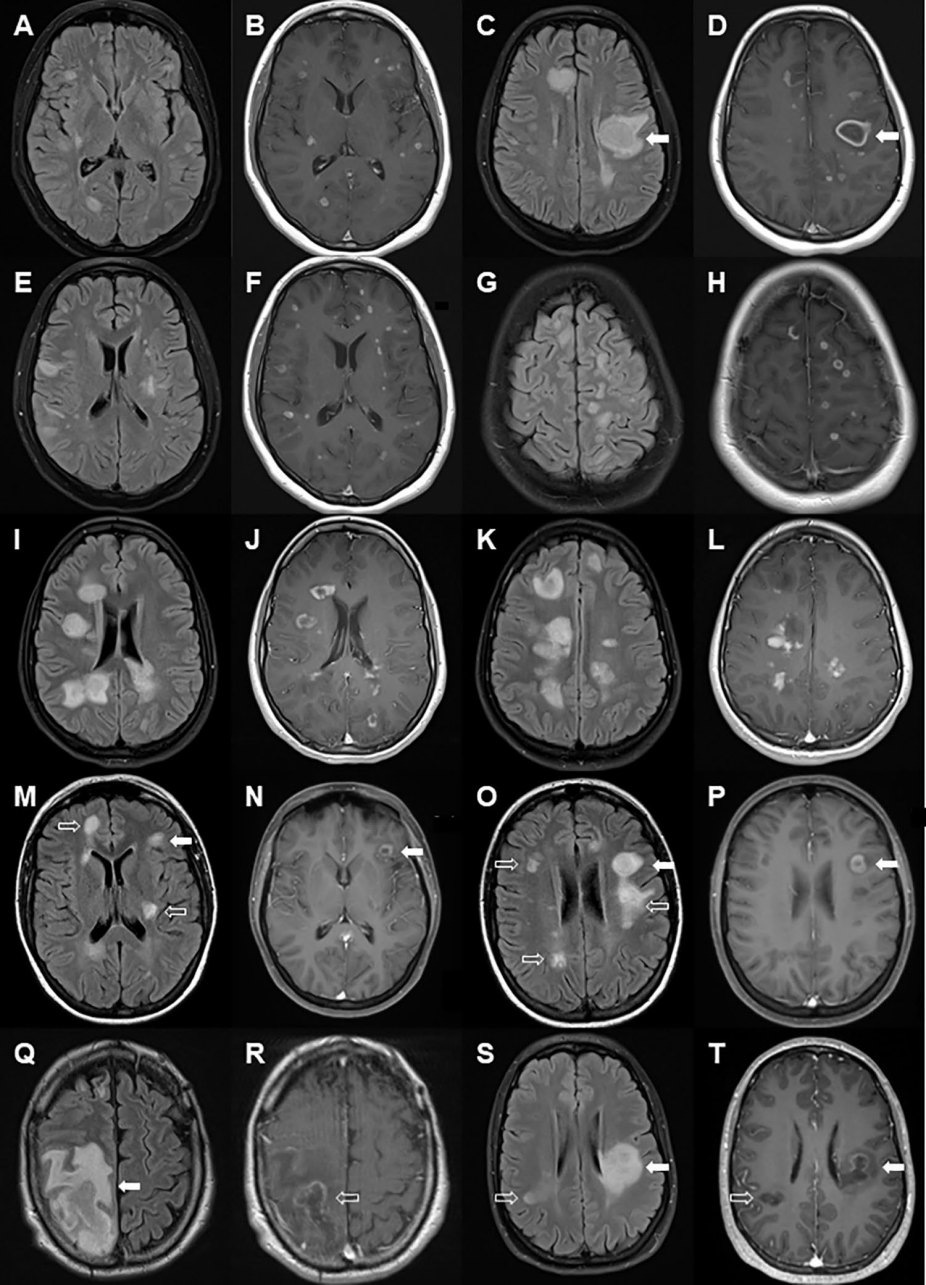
MRI of ADEM-like and tumefactive MS cases. Images are paired (matched slices) with FLAIR images in first and third vertical panels and Gd-enhanced T1 weighted sequences in second and fourth vertical panels. Case of ADEM-like lesions with small and large lesions which all show Gadolinium enhancement (A–D), one larger lesion (D arrow) shows ring-enhancement with central hypointensity and peri-lesional hypointensity with surrounding oedema. Case of ADEM-like lesions with a multitude of smaller lesions all of which show either homogeneous or ring enhancement (E–H). Case of ADEM with multiple large lesions showing both ring enhancement and heterogeneous enhancement. Case of ADEM-like lesions showing multiple small and large lesions (M–N). Some lesions are non-enhancing, with some having central hypointensity on T1 sequences (M and O open arrows) whilst other lesions show ring-enhancement (open arrows). Case of recurrent tumefactive MS showing large incomplete ring-enhancing lesion with central hypointensity and surrounding oedema (solid arrow) with mass effect (S and T). Case of tumefactive MS with a large incomplete ring-enhancing lesion (solid arrow) with central and perilesional hypointensity and a second non-enhancing lesion (open arrow) with central hypointensity (S and T)
